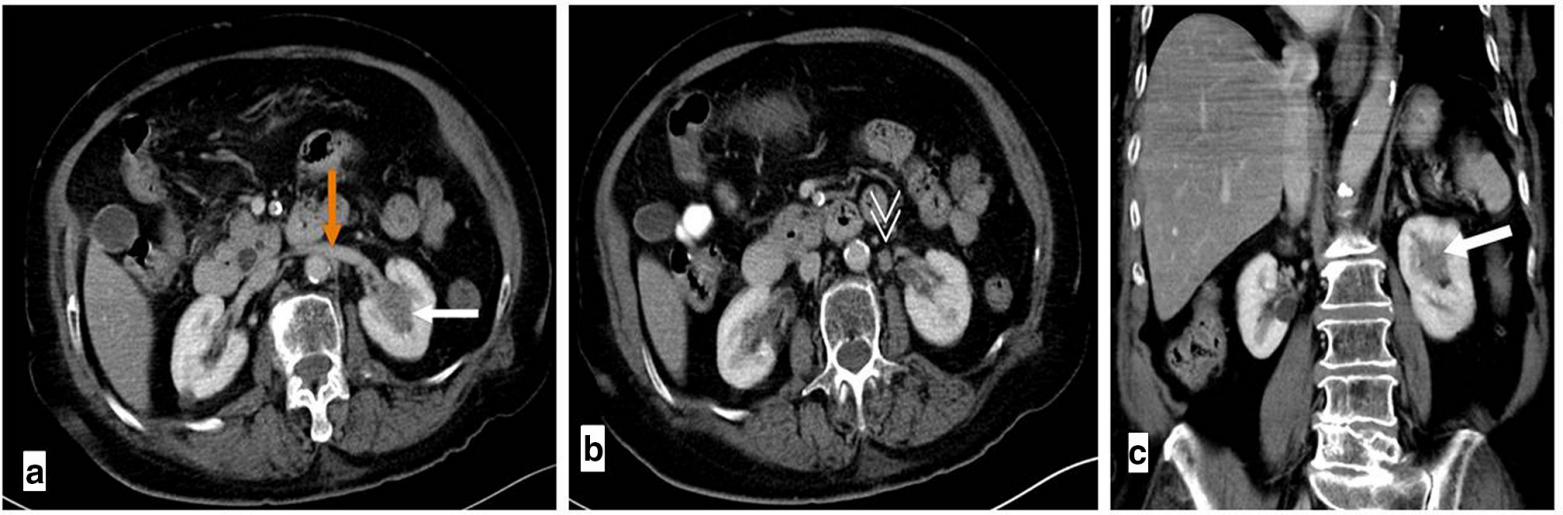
Contrast-enhanced axial (a, b) and coronal (c) CT scan images showing a small ill-defined non-enhancing mass in the left renal sinus (white arrows). Small loco-regional lymph node (white arrowhead in B) adjacent to the patent left renal vein (orange arrow in A). Abbreviations: CT, computed tomography.
